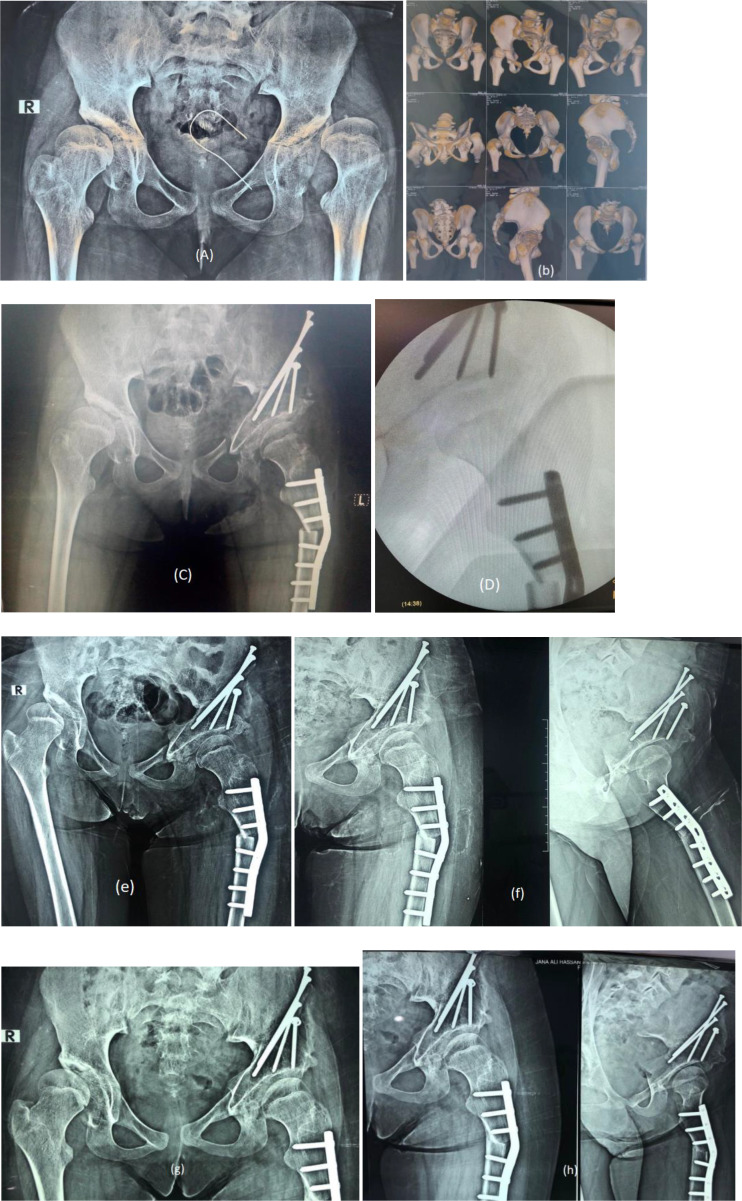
(a) Preoperative plain X-ray AP pelvis of 14 years old female with left hip dislocation and acetabular dysplasia, (b) preoperative CT scan, (c, d) immediate postoperative plain X-ray and intraoperative X-ray of femoral varus shortening osteotomy with TPO, (e, f) 1 month postoperative X-rays, (g, h) 6 months postoperative X-rays.
